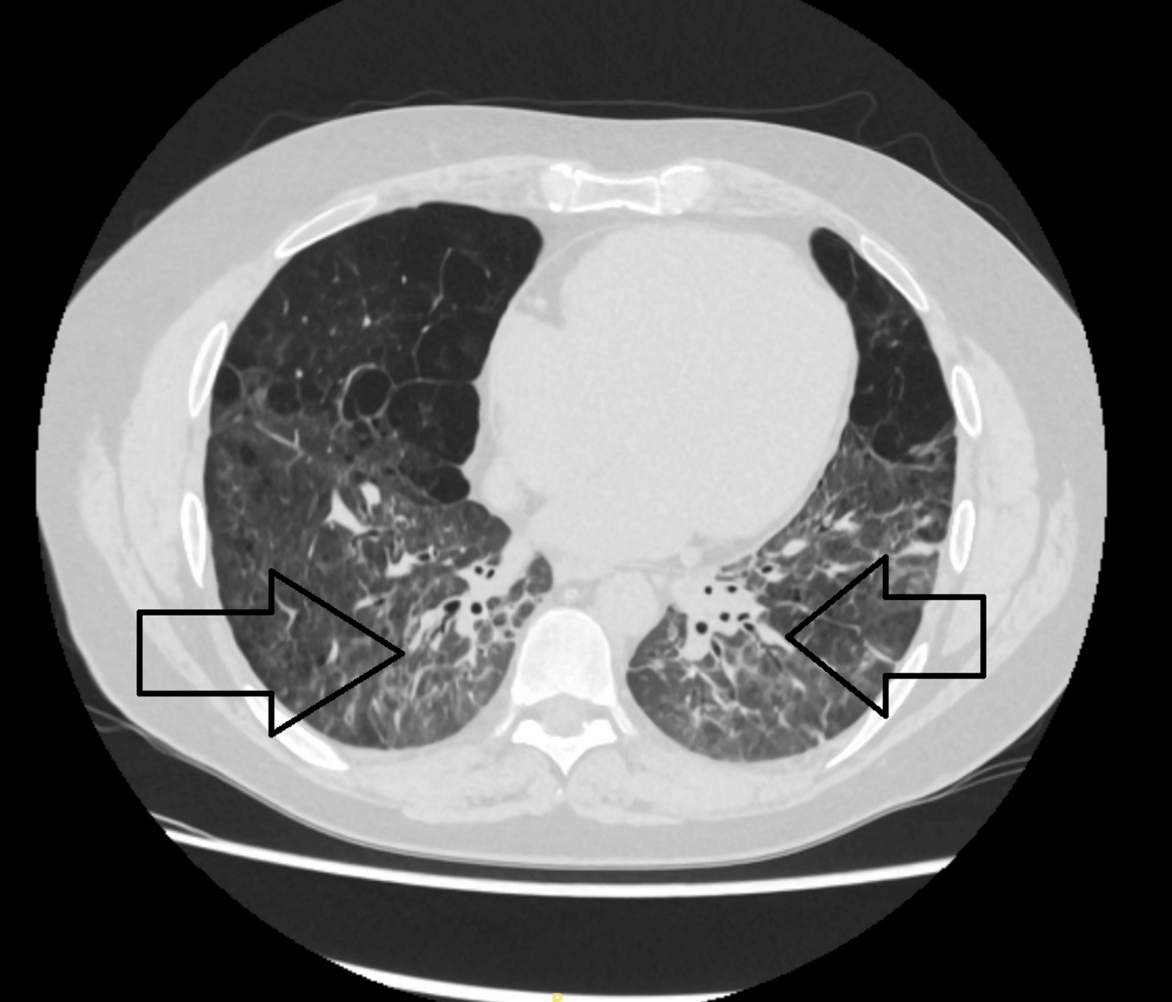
Axial CT chest (May 2025) shows near-complete resolution of lung infiltrates after anakinra therapy, with minimal residual fibrotic changes (black arrows). Improvements reflect IL-1 receptor antagonist treatment for MAS secondary to AOSD. CT: computed tomography; IL-1: interleukin-1; MAS: macrophage activation syndrome; AOSD: adult-onset Still’s disease
